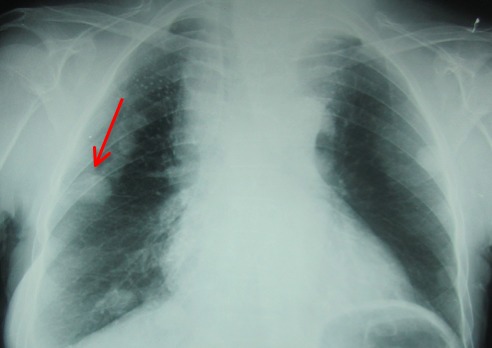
Radiographie du thorax en pré-opératoire montrant les métastases pulmonaires
